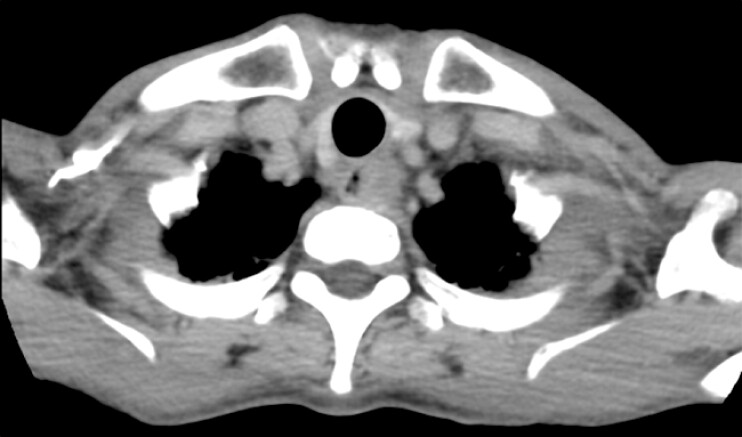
A CT scan revealed an image showing the cervical esophagus occupied by esophageal cancer. CT, computed tomography.
